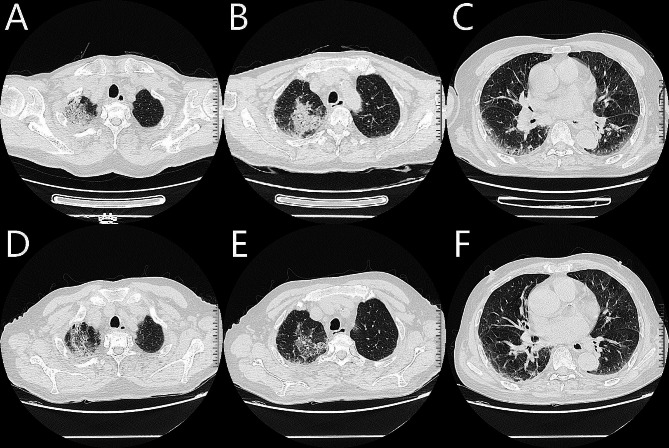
Comparison of chest HRCT scans before and after treatment. (A-C) Following admission on September 22, 2022, a chest HRCT revealed multiple flocculent density enhancement shadows in both lungs, with the right upper lobe being particularly prominent. (D-F) Following treatment with corticosteroids (methylprednisolone 40 mg, once every 12 h, for 3 days), the chest HRCT demonstrated a decrease in multiple flocculent density enhancement shadows in both lungs compared to the pre-treatment scan, as well as slight bronchiectasis in the apical segment of the right upper lobe
